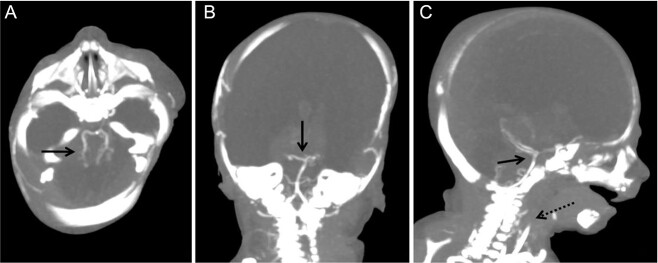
Axial (A), coronal (B), and sagittal (C) brain postmortem computed tomography angiography (PMCTA) (maximum intensity projection, reconstructions) showing a full opacification of the vertebral arteries and basilar trunk with both posterior inferior cerebellar arteries (black arrows), and the absence of opacification of the cervical terminal part of both internal carotids (black spotted arrow). Anterior and middle cerebral arteries are not visible, as well as intra-cerebral veins.
